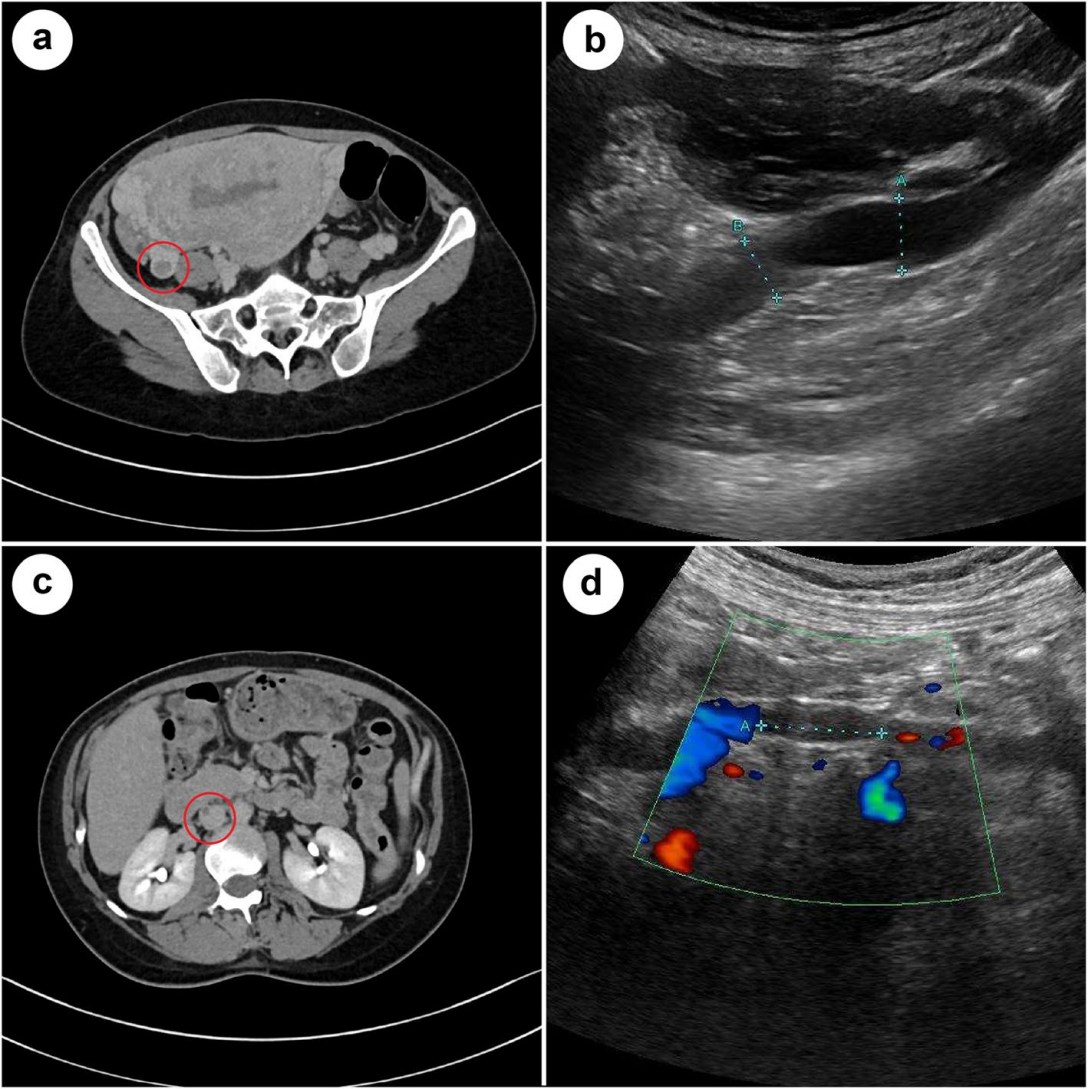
Computed tomography and trans-abdominal ultrasonography of right ovarian vein thrombosis on the days of detection (a, b) and extension to the inferior vena cava (c, d)
